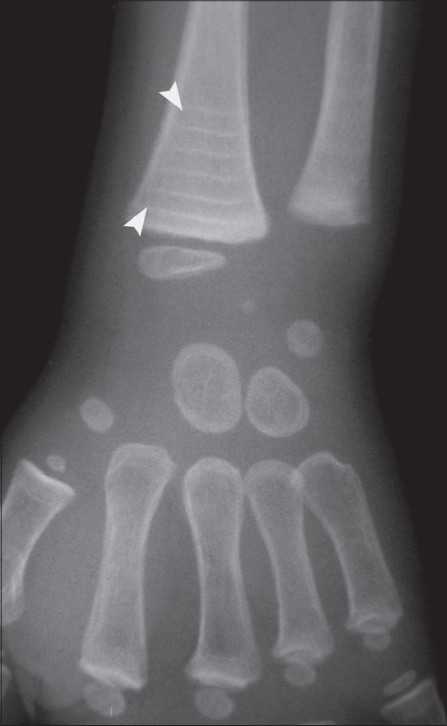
Posteroanterior radiograph of the wrist shows zebra lines (white arrow heads)
